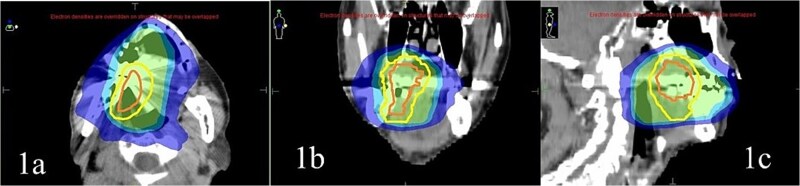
Irradiation area; (a) axial view, (b) coronal view, (c) sagittal view. Gross target volume (GTV) (orange line): Region of visible lesion and tumor extension identified by FDG-PET, contrast-enhanced CT, and magnetic resonance imaging. Clinical target volume (CTV): Defined by adding a 5-mm margin to the GTV. PTV (Yellow line): Defined by adding a 5–10 mm margin to the CTV. Regions receiving 95%, 80%, and 50% of the prescribed dose are indicated in light green, light blue, and blue, respectively. GTV, Gross target volume; CTV, Clinical target volume; PTV, Planning target volume; FDG-PET, Fluorodeoxyglucose positron emission tomography.
